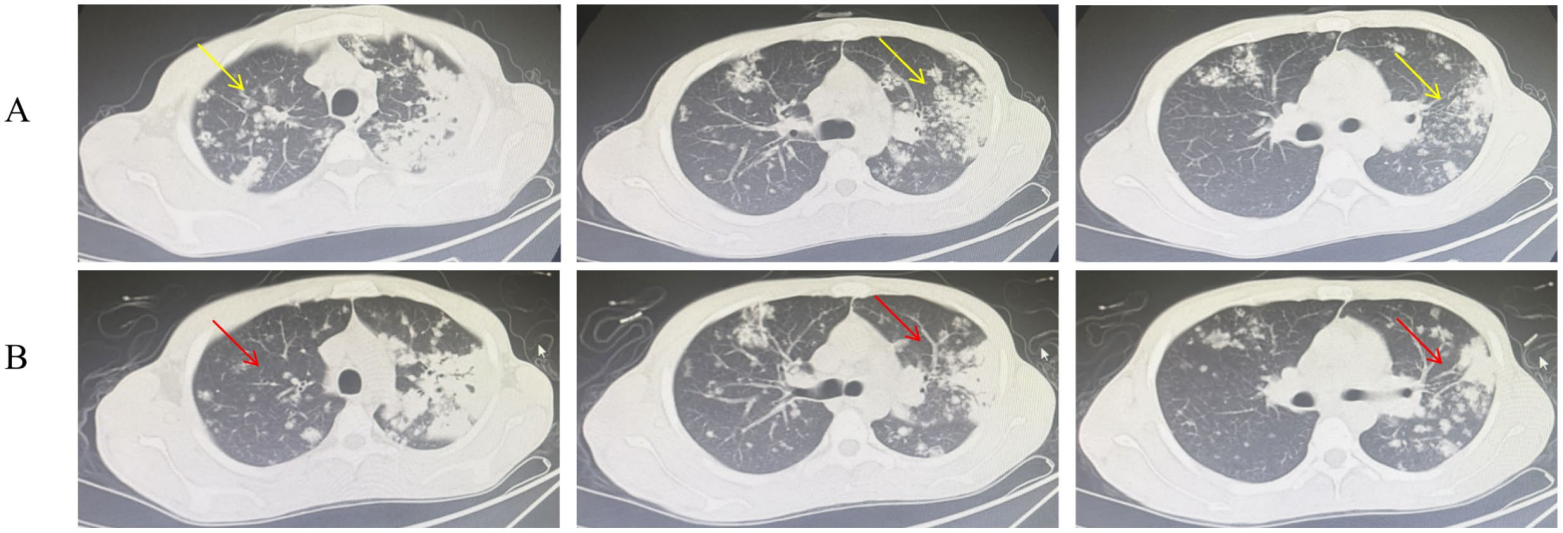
Chest CT scans before (A) and after (B) 4 weeks of anti-tuberculosis therapy. (A) Pre-treatment image shows apical-predominant infiltrates with a cavitary lesion (yellow arrow). (B) Post-treatment image shows resolution of infiltrates and reduction in cavity size (red arrow).
